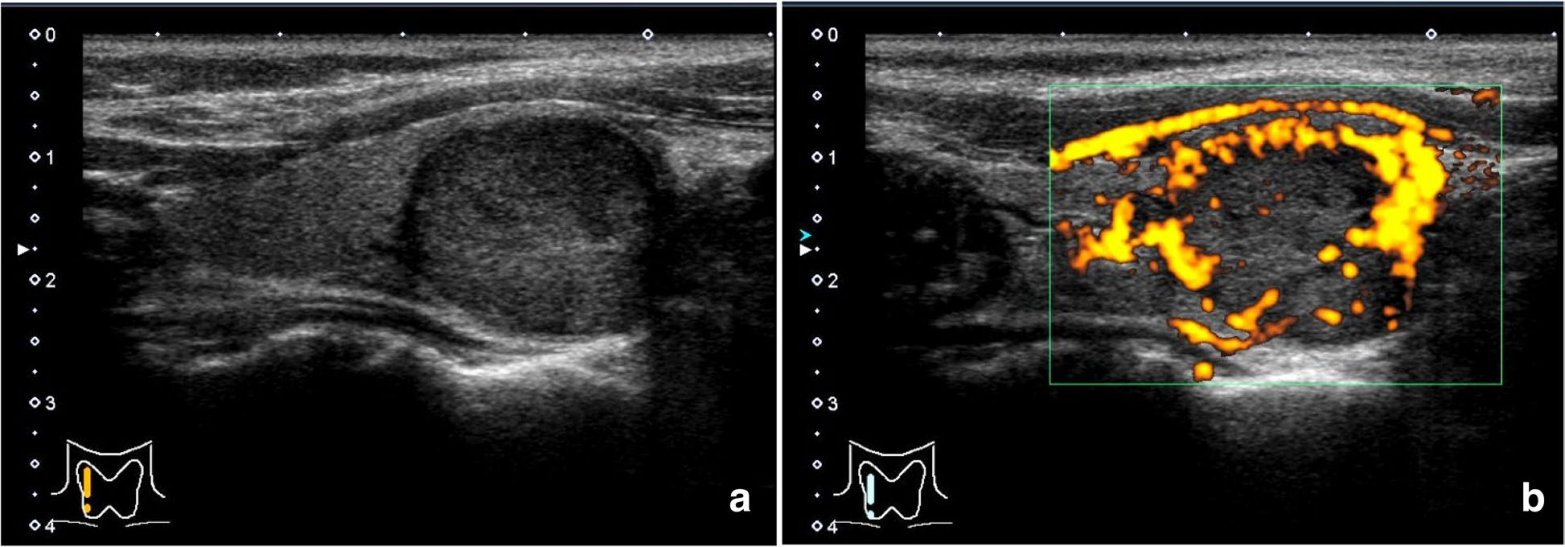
A well-defined, hypoechoic, and oval mass is present in the right lobe (a). Doppler imaging reveals increased peritumoral blood flow (b) (B-mode)
